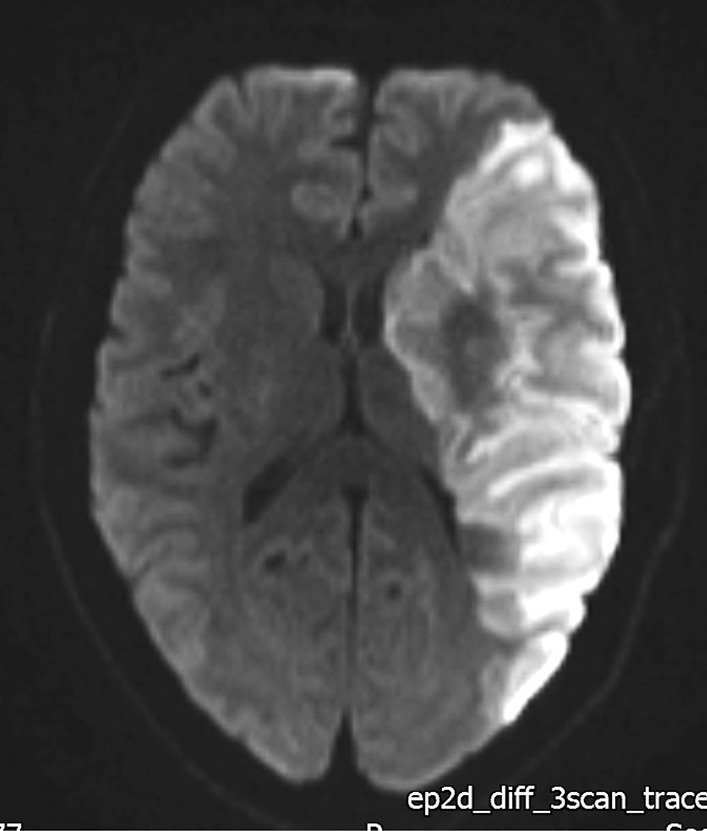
Diffusion-weighted MRI Brain showing restricted diffusion in the region of the left middle cerebral artery
